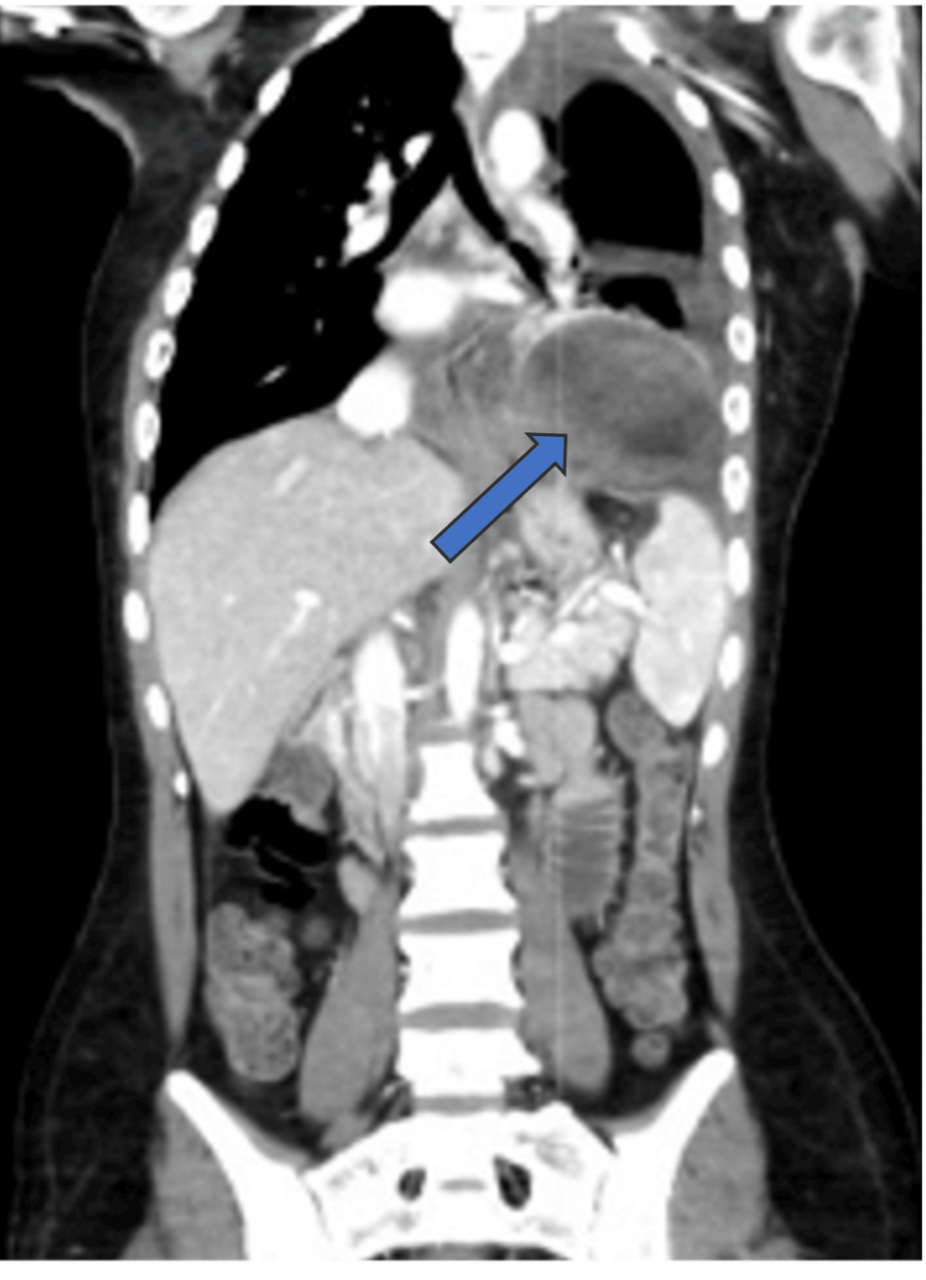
CT chest and abdomen (July 11, 2024) showing a 2.5 cm defect in the left hemidiaphragm (arrow) with herniation of the gastric fundus into the pleural cavity, associated with pleural effusion and atelectasis.
